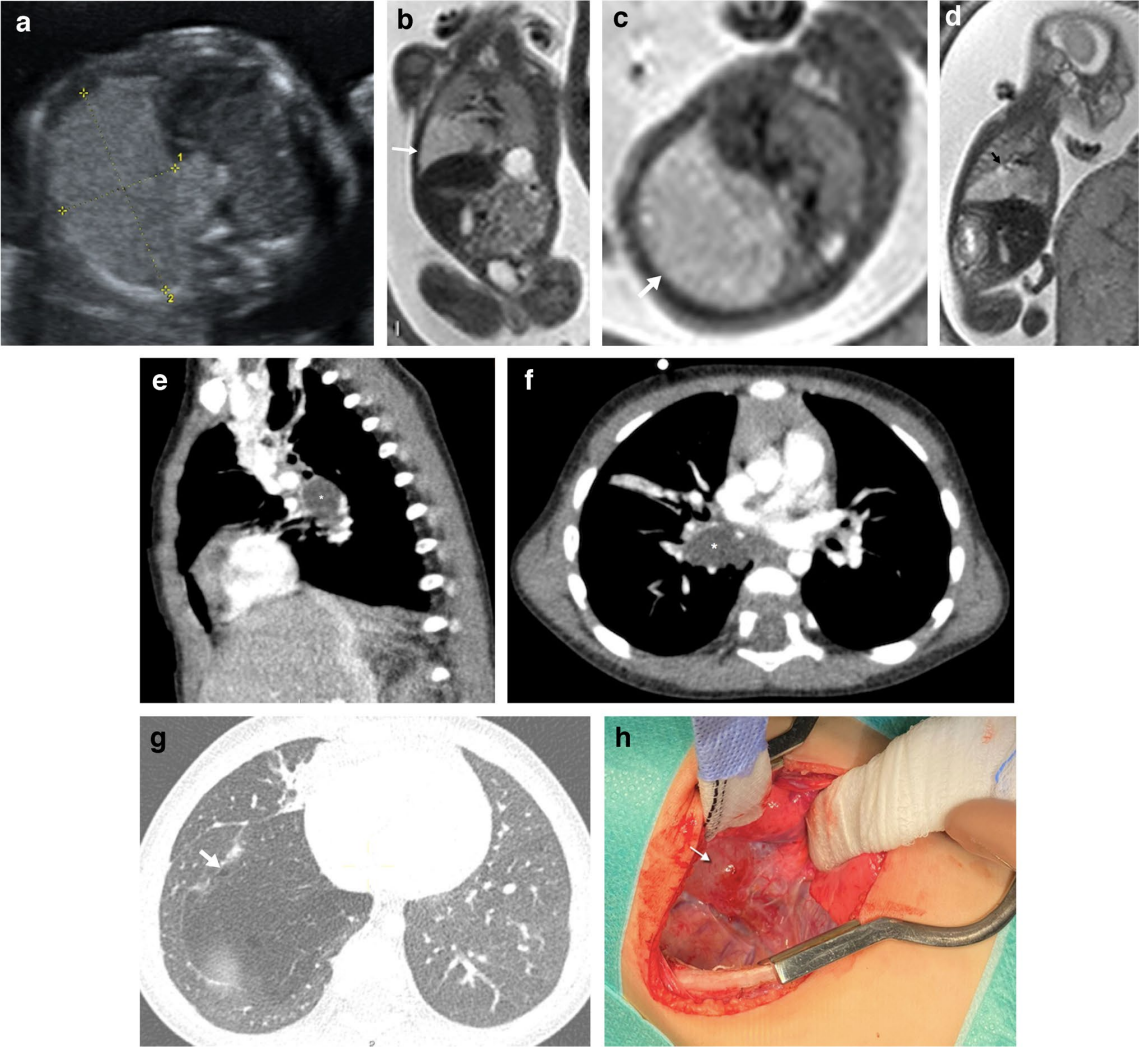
a–d Bronchogenic cyst and congenital pulmonary airway malformation in a 20-week gestational age male fetus. a An axial ultrasound image of the chest shows a large homogeneous hyperechoic lesion in the right lung base (*calipers*). b–d Single-shot T2 coronal (b), axial (c) and sagittal (d) magnetic resonance images show a large homogeneous lesion in the right lung base of high signal intensity on T2 (*white arrows in *b and c), with small cysts in the periphery (*black arrow in *d). No abnormal feeding vessel or hilar cystic lesion was identified. e–g Sagittal (e) and axial computed tomography images on mediastinal (e, f) and lung window (g) settings obtained at 6 months of age show a homogeneously hypodense cystic lesion in the right pulmonary hilum (*asterisks*) with atelectasis in the middle lobe and hyperinflation in the left lower lobe, with small peripheral cysts (*arrow in *g). h Photograph obtained at surgery demonstrates a bronchogenic cyst (*arrow*) associated with congenital pulmonary airway malformation
